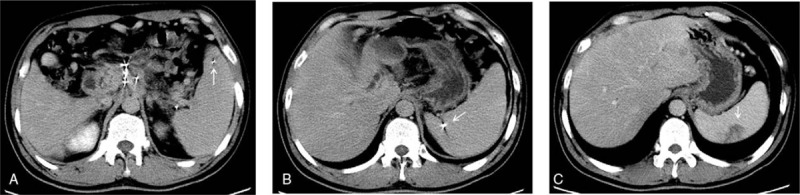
Iodine-125 seeds migrating to spleen and leading to spleen infarction. (Left and Center) The migrated iodine-125 seeds were found in spleen on the CT scans during the follow-up of post-implantation (arrow). (Right) Infarction of spleen resulted from seeds migration was confirmed on the enhanced CT scans (arrow).
